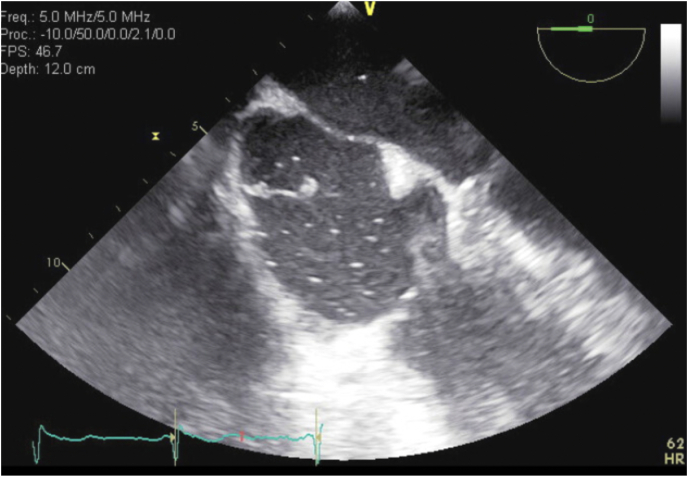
Transesophageal echocardiogram (TEE) confirming vegetation on the Eustachian valve, likely from *E. coli* ESBL.
